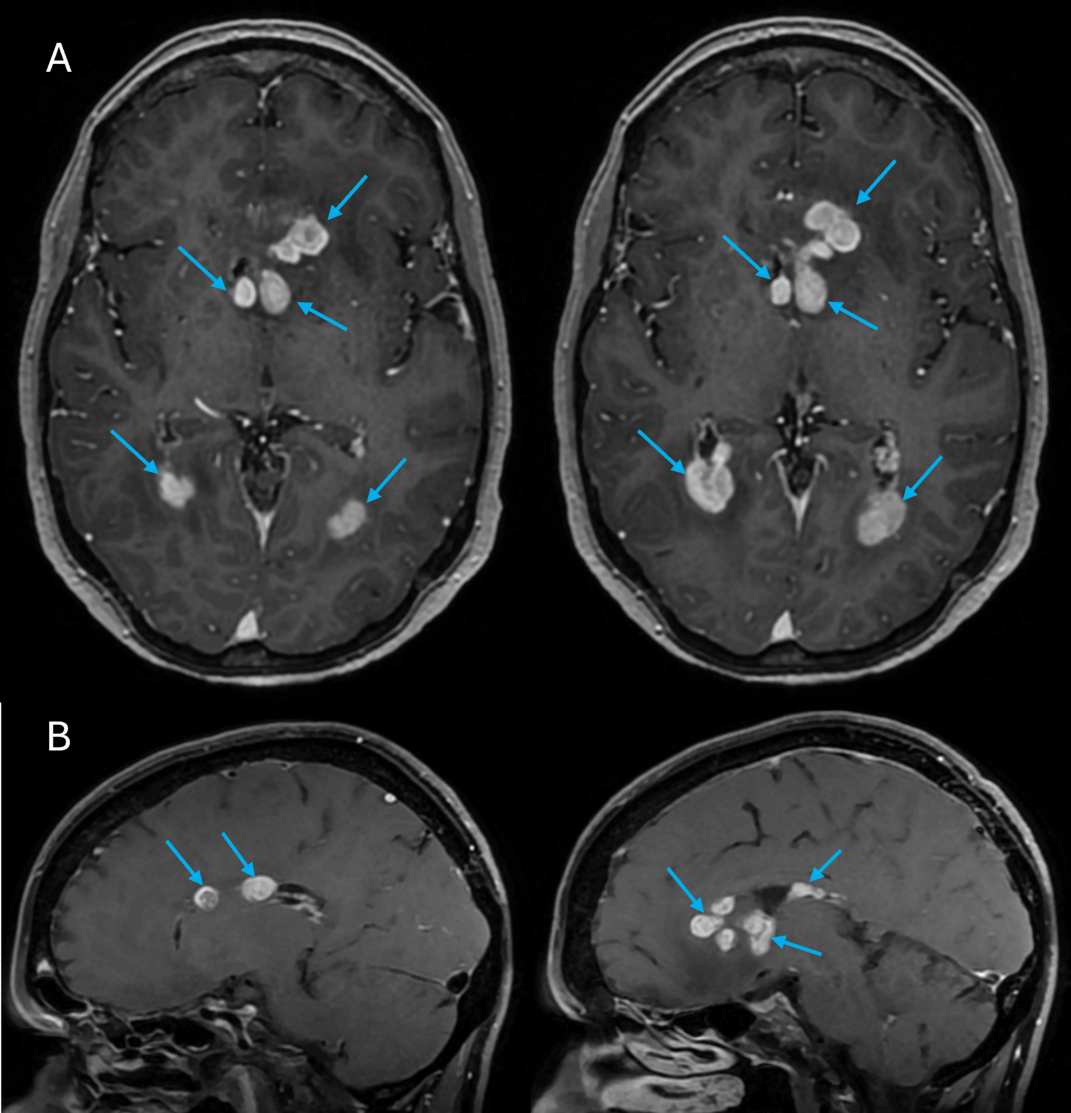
MRI scans showing interval progression of the intraventricular lesions (A) Axial and (B) Sagittal T1 MRI scans with contrast showing progression of the ependymal enhancement of the lateral ventricles bilaterally (blue arrows)
